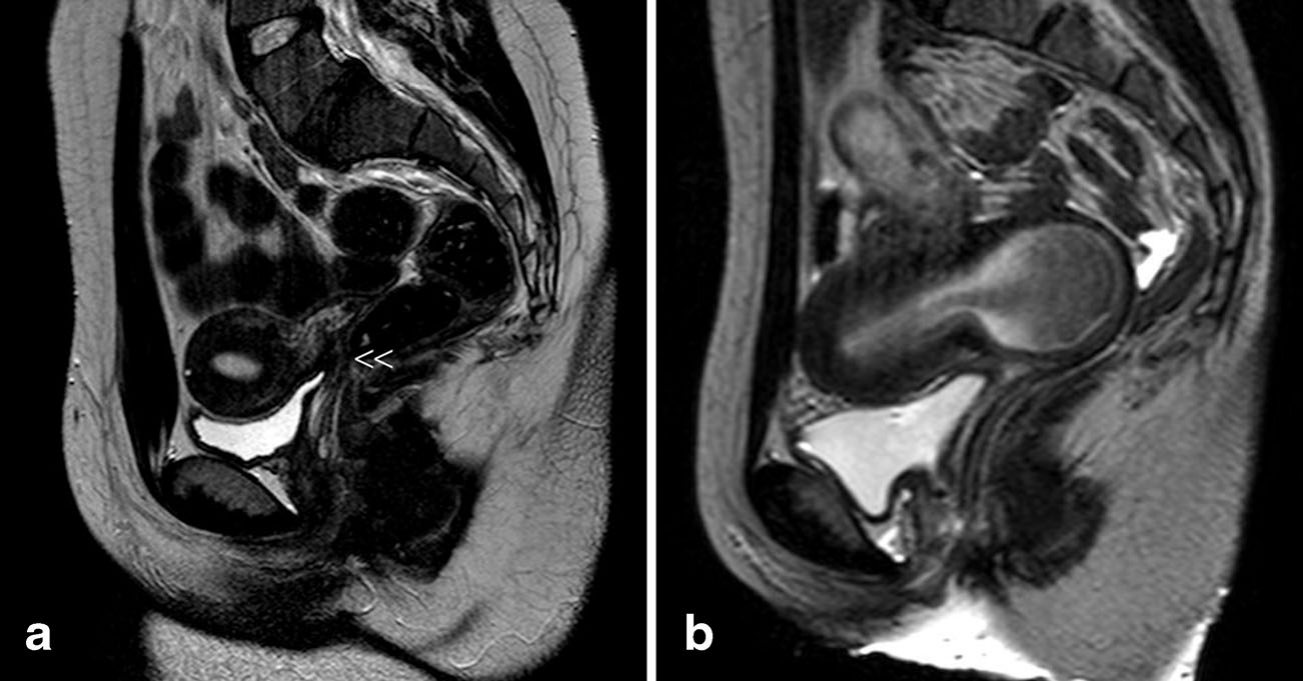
Cases with vaginal or cervico-vaginal atresia or agenesis and normal uterine corpus. a T2-weighted MR image in a 20-year-old patient with complete cervico-vaginal atresia. Medial sagittal section showing the uterus and cervico-vaginal atresia (<<). b T2-weighted sagittal plane in the other case with vaginal atresia and haematocervicometra (courtesy of Dr. MJ. Lázaro, Oviedo)*Vaginal segmentary atresia and transverse vaginal septum* correspond to a transverse constriction or septum that is perforated or imperforated. There may be no symptoms until puberty when the haematocolpos forms and causes episodes of pelvic pain and primary amenorrhoea similar to those observed with vaginal atresia [49, 50]. The examination, abdominal or TRU and specially MR allow the diagnosis and help on the surgical evacuation of the haematocolpos. Uterus, fallopian tubes and ovaries are usually normal.4.*Gubernaculum dysfunctions*: These cases are typified by accessory and cavitated uterine masses (ACUMs) with an otherwise normal uterus [51–53]. HSG will show a normal endometrial cavity and 3D-US and especially MR allow the right diagnosis.5.*Anomalies of the cloaca and urogenital sinus (including congenital vagino-vesical fistulas)*: This category includes cases as simple as the imperforate hymen due to a persistent urogenital membrane together with blind hemibladder [54], bladder duplication [55, 56], bilateral single system ectopic ureters opening into the vestibule or a vaginalised urogenital sinus with bladder agenesis or hypoplastic bladder [57–59] or congenital vesico-vaginal or vesico-uterine fistulas (pseudofistula with menuria [60, 61]) and cloacal exstrophy [62, 63] (Fig. 8). Diagnosis can be made with the physical examination together with TRU, IVP (eventually, retrograde pyelogram), cystouretroscopy, cystography, CT, and specially MR, or abdominal US for prenatal diagnosis [56, 64].
